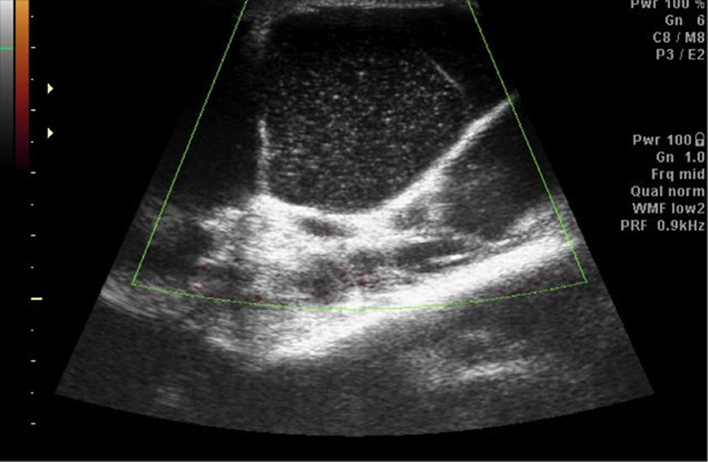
Ultrasound image of the knee showing multiple loculated cystic lesions involving the subcutaneous planes and the popliteal fossa with diffuse internal echoes and septations. No internal vascularity is seen.
